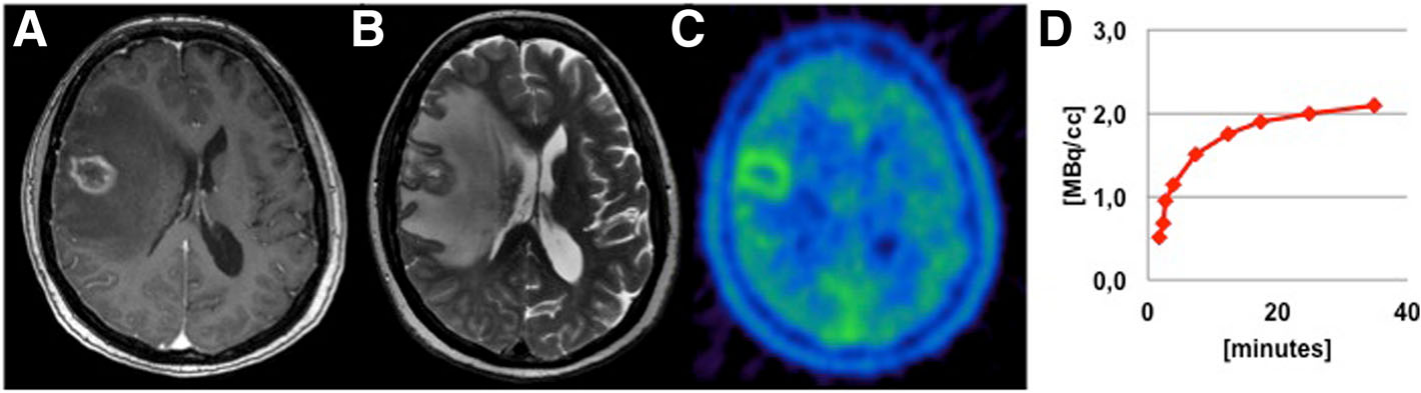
MRI and ^18^F-FET PET findings in a radiation-induced lesion after focused high dose radiotherapy (Patient nr. 18). A ring-like contrast enhancement in the T1-weighted images (a), as well as an extensive edema in the T2-weighted images is seen (b). Both a marginal focal ^18^F-FET uptake (TBR_max_ 1.4, TBR_mean_ 1.3) and increasing TACs can be observed in static and dynamic PET analysis (c, d)
